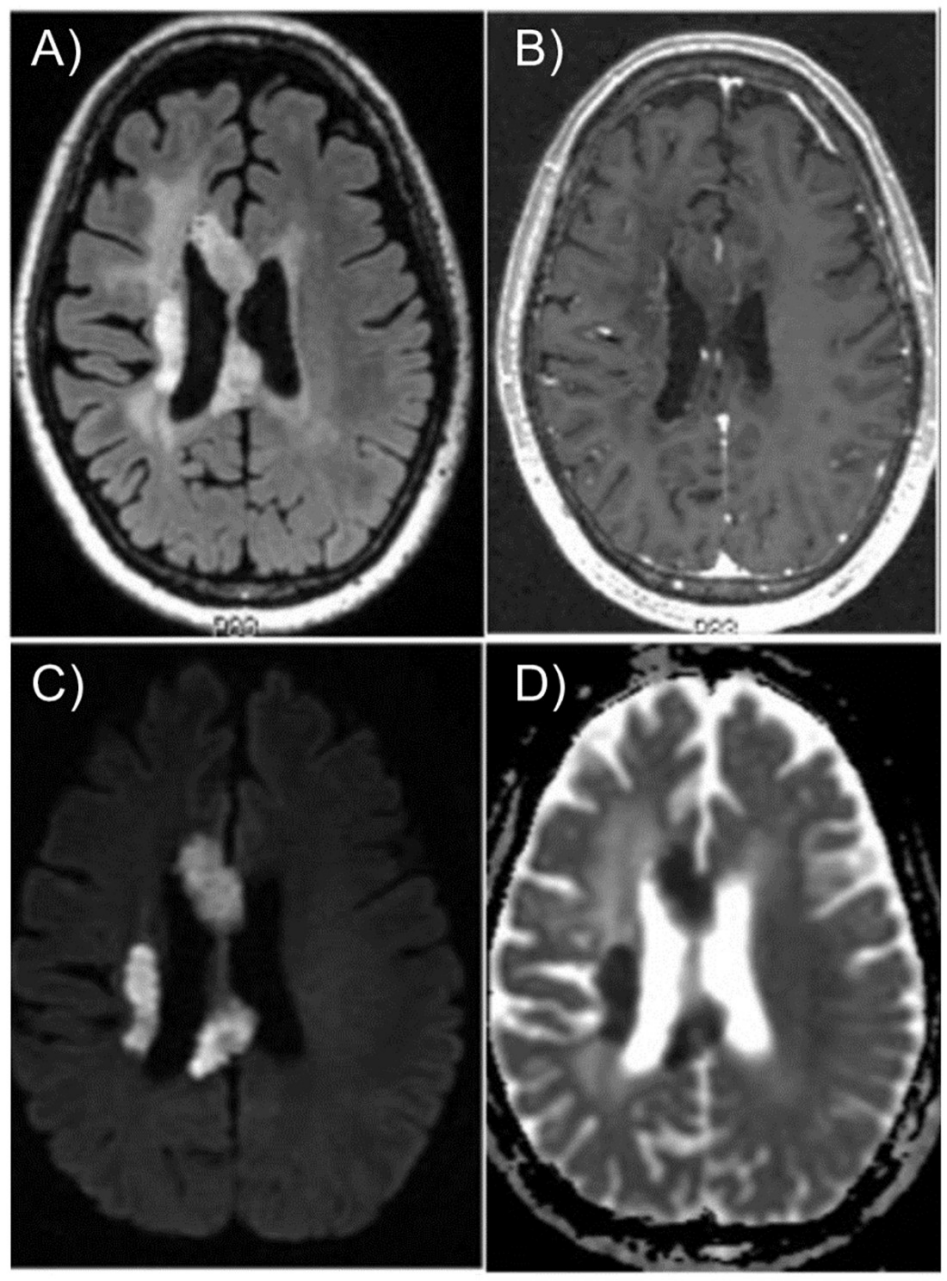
Example BRIA lesion. Appearance of BRIA in a 65-year-old male two months after starting bevacizumab for recurrent right parietal glioblastoma on a) FLAIR, b) T1 post-contrast, c) DWI, and d) ADC. Abbreviations: FLAIR, fluid-attenuated inversion recovery; DWI, diffusion-weighted imaging; ADC, apparent diffusion coefficient.
